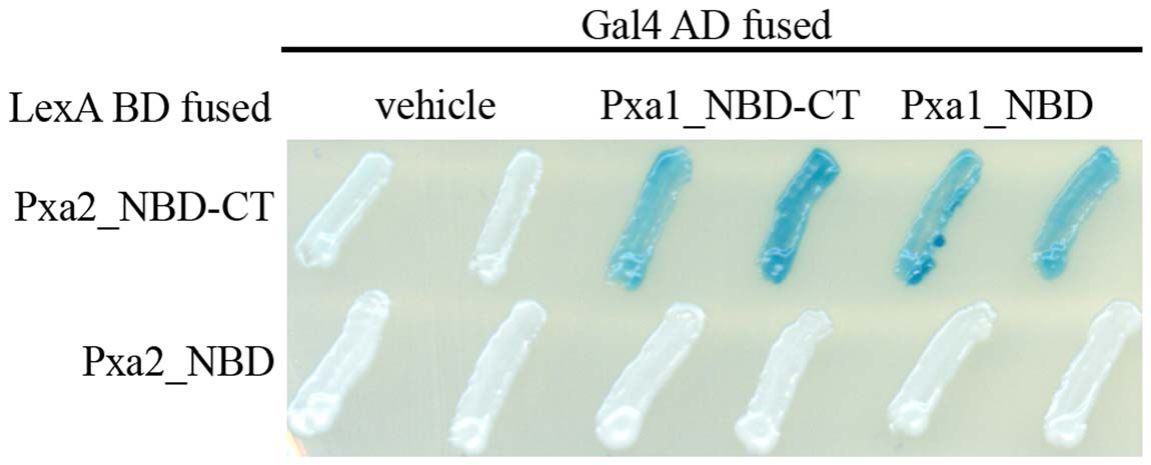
Two-hybrid analysis of interactions between Pxa2_NBD-CT (or Pxa2_NBD) and Pxa1_NBD-CT (or Pxa1_NBD). The EGY48 (pSH18-34) reporter strains that express the indicated hybrid proteins (with the Pxa2 fragment fused to LexA BD and the Pxa1 fragment fused to Gal4 AD) were analyzed for β-galactosidase activity on an X-gal plate. Plates were incubated for 16 h at 30°C. Blue indicates the existence of a protein-protein interaction between hybrid proteins.
